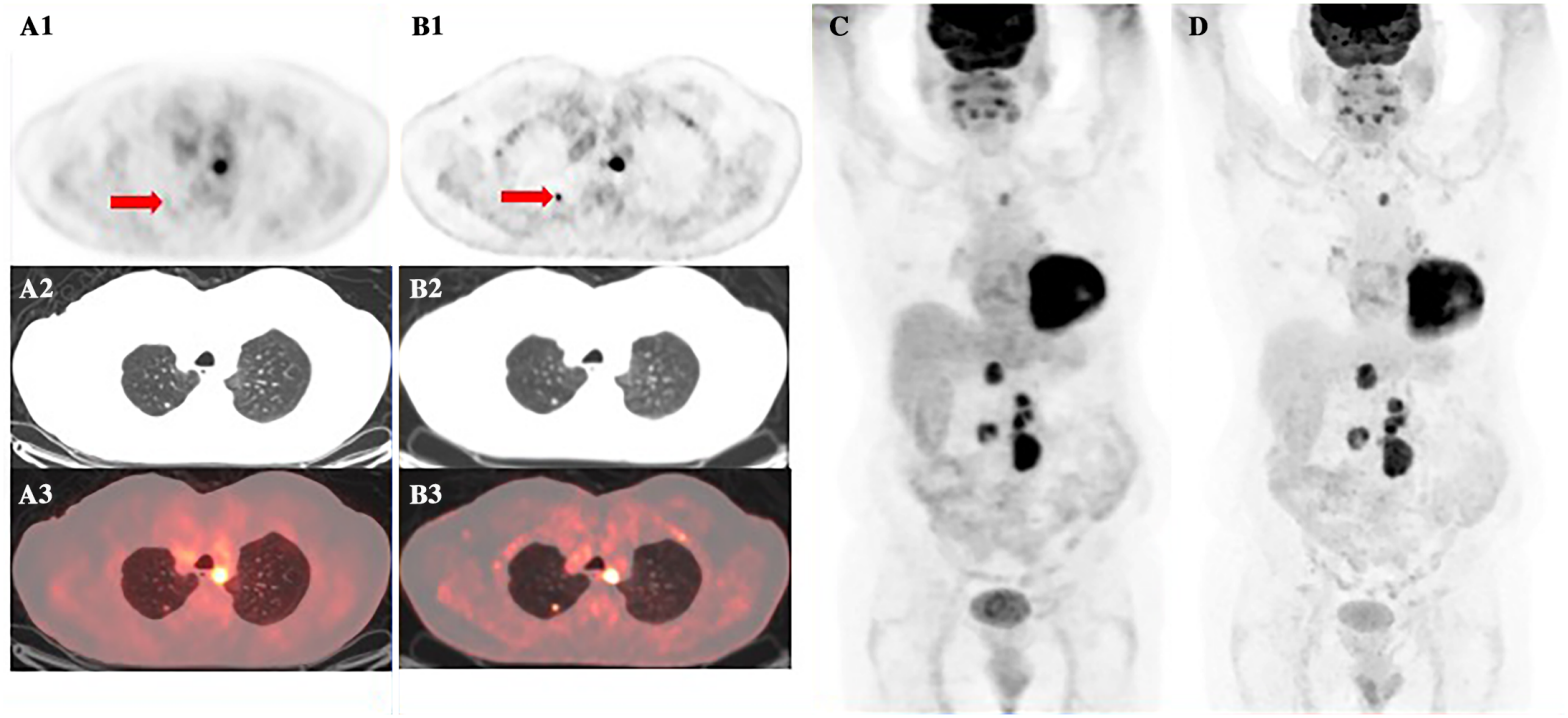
D690 PET/CT vs DMI PET/CT: DMI showed a significant increase in SUV_max_ (red arrow). 79 years-old man with kidney cancer who performed PET/CT for evaluation of response to therapy. DMI images were acquired 34 minutes after standard PET/CT acquisition and 104 minutes after ^18^F-FDG injection. A1) D690 PET; A2) D690 CT; A3) D690 fused image; B1) DMI PET; B2) DMI CT; B3) DMI fused image; C) D690 MIP; D) DMI MIP.
